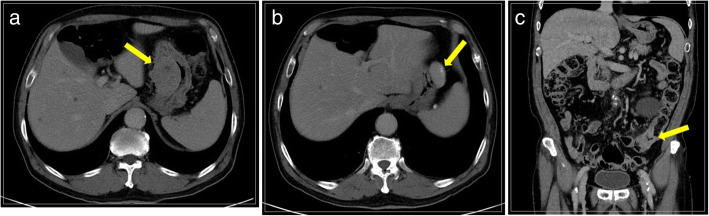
Computed tomography scan showing the presence of two submucosal tumor lesions in the stomach and wall thickening of the descending colon. a Mass on the lesser curvature of the stomach. b Mass on the anterior wall of the stomach. c Wall thickening of the descending colon. Yellow arrow indicates the mass
